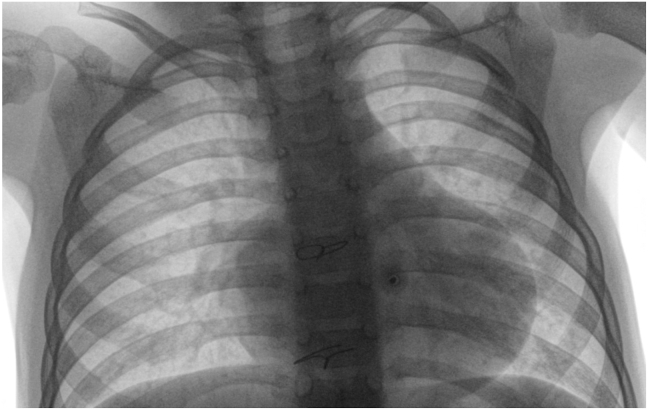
Radiographic image after occluder device placement and pulmonary artery banding in a child with multiple ventricular septal defects.
